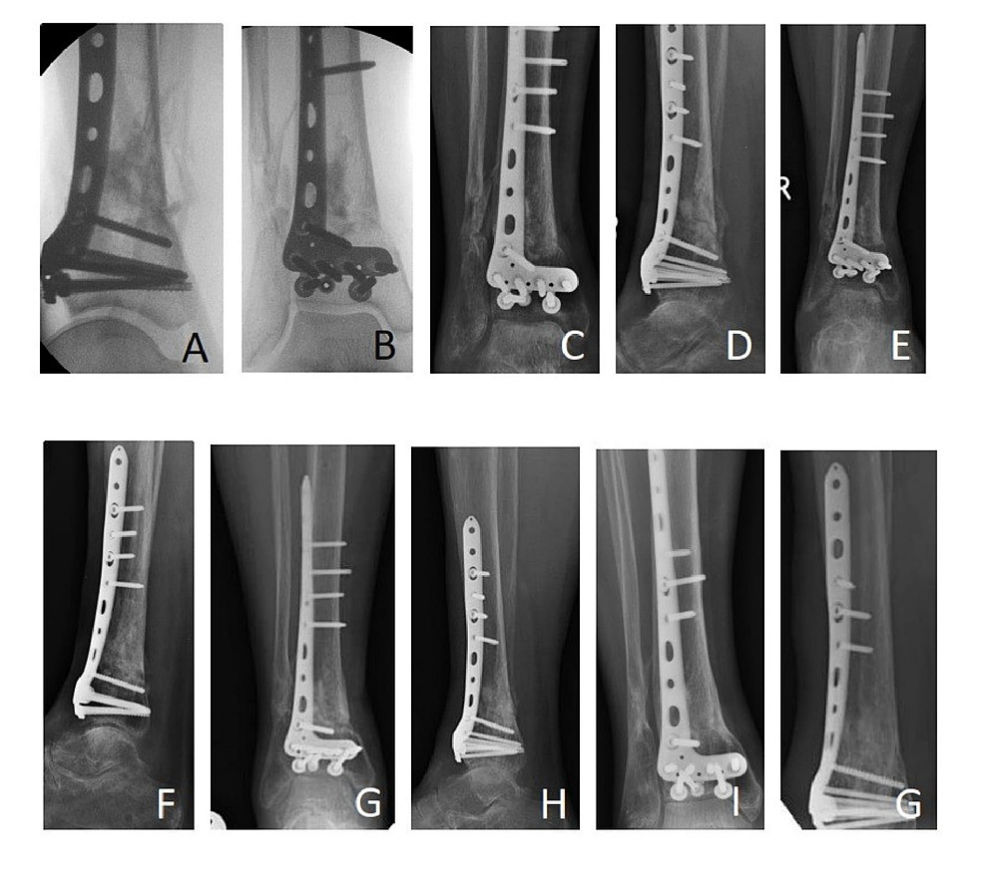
Radiological signs of Cerament-G remodelling seen on X-ray images taken at different stages of healing. A&B: at time of surgery; C&D: at six weeks postoperatively; E&F: at three months postoperatively; G&H: at six months postoperatively; and I&J: at the final follow-up.
